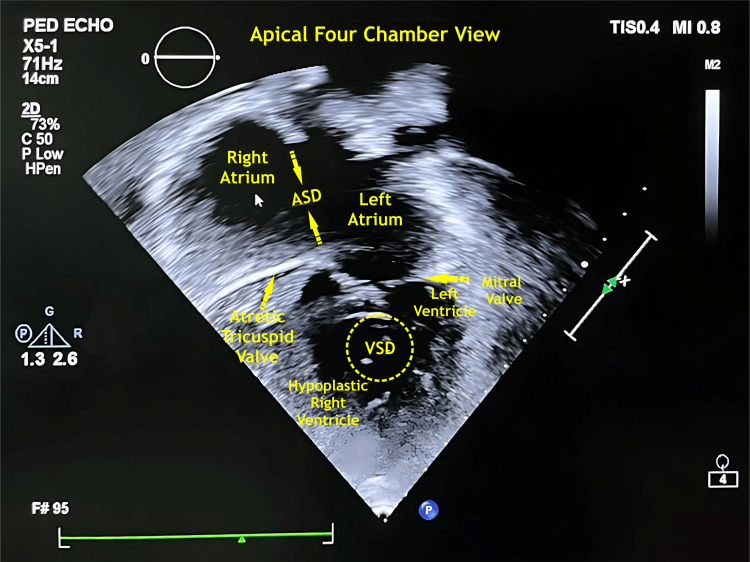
2D echocardiography apical four-chamber view ASD - atrial septal defect, VSD - ventricular septal defect
